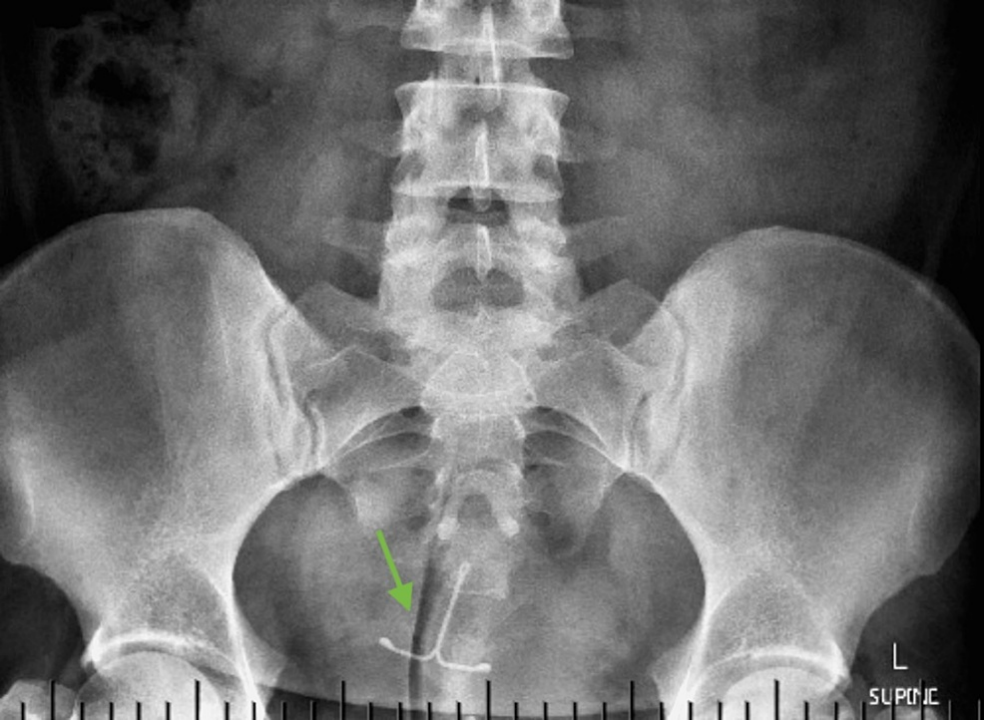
Erect X-ray of the patient revealing the LNG-IUD “inside” the pelvic cavity in an “upside down” position in the vicinity of the uterus. LNG-IUD: levonorgestrel-releasing intrauterine device
